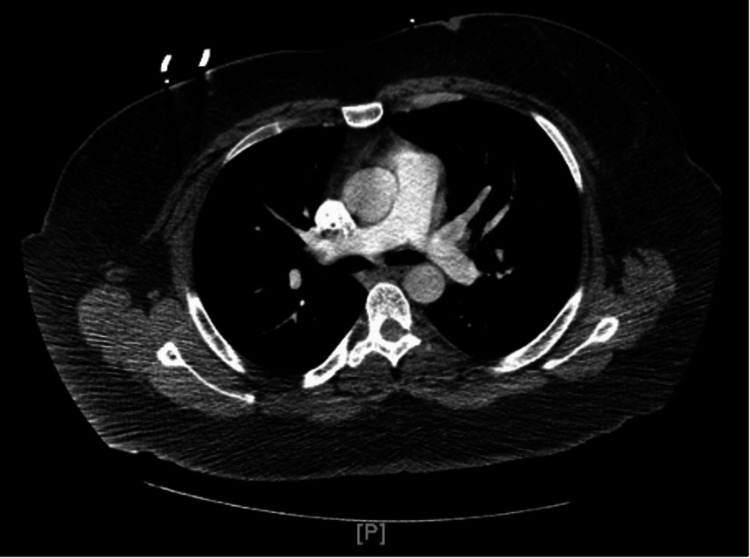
The Computed Tomography Angiogram (CTA) scan of the chest showed no evidence of pulmonary embolism
